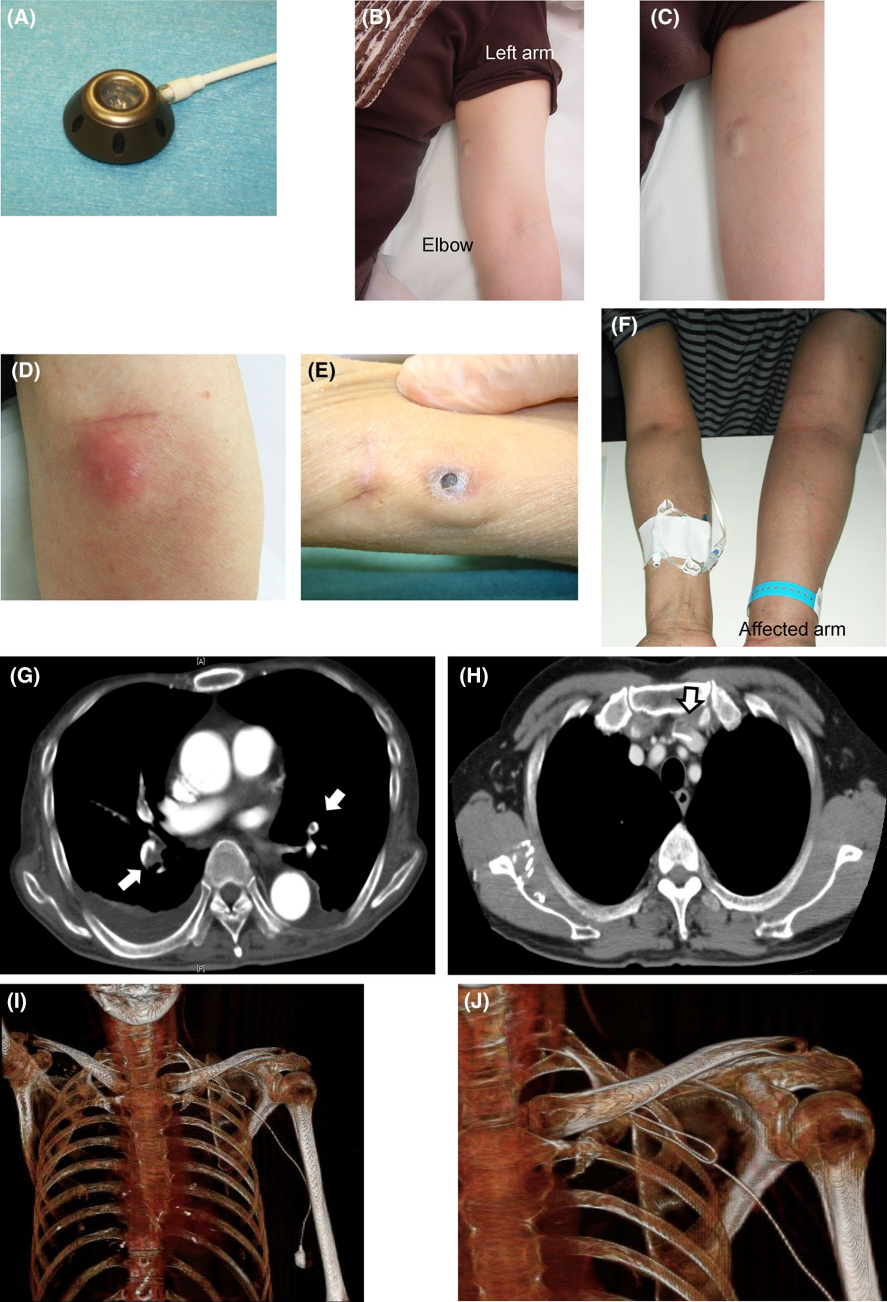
Subcutaneously implanted UACVP and representative manifestations of UACVP-related complications. a Titanium Vital Port system. b, c CVP was implanted on the ulnar side in the upper arm. d Port pocket infection with erythematic induration. e Skin dehiscence leading to the exposure of a subcutaneous CVP. f Venous thrombosis resulting in swelling of the left upper extremity. The patient underwent systemic anticoagulant therapy via the right peripheral blood vessel. g Bilateral pulmonary embolism. h Thrombus detected around the catheter in the subclavian vein. i, j Catheter dislocation on three-dimensional computed tomography
